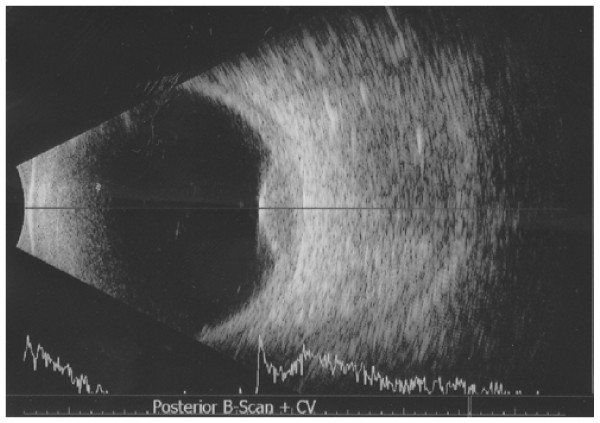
Ultrasound of the left eye. Combined A-scan and B-scan ultrasound showing an acoustically hollow dome-shaped lesion, which demonstrated a medium to low reflectivity, and a maximum height of 3.15 mm.
